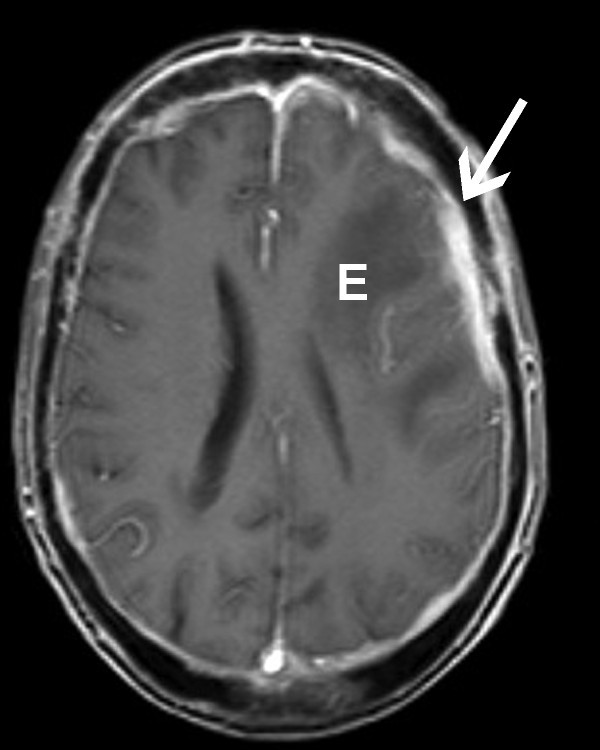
MRI with contrast. Vasogenic edema - E. Nodular dural thickening - arrow.
